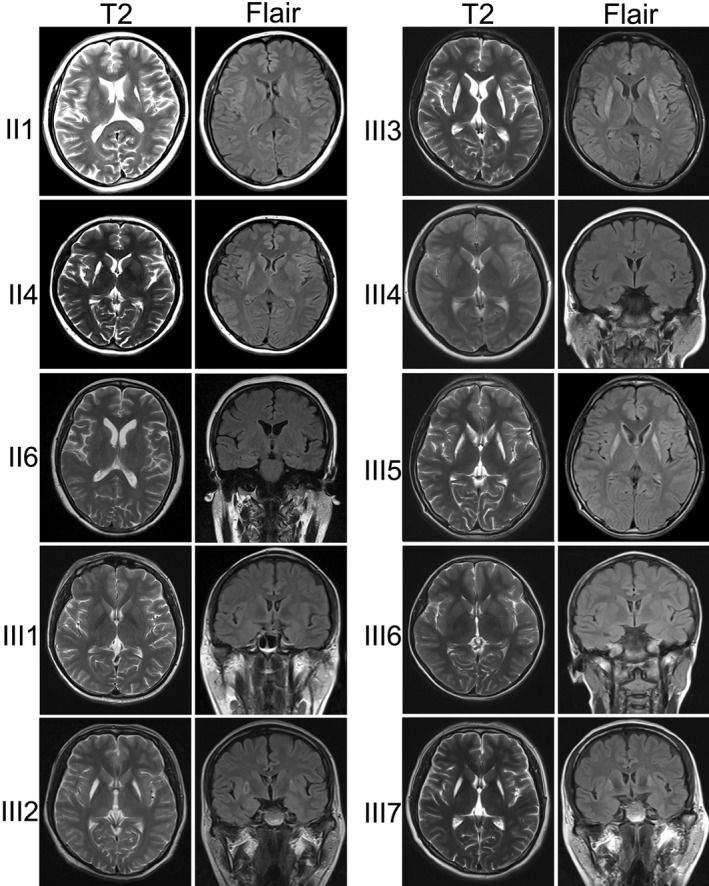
Brain MRI findings of family individuals. Magnetic resonance images of patients showed high‐intensity signals in the bilateral putaminal areas on both T2‐weighted (T2) and fluid‐attenuated inversion recovery (FLAIR) images in male patients III2, III3, III5, and III7; unilateral putaminal areas in female patients II1 and II4; and normal cerebral structure in other female individuals II6, III1, III4, and III6
